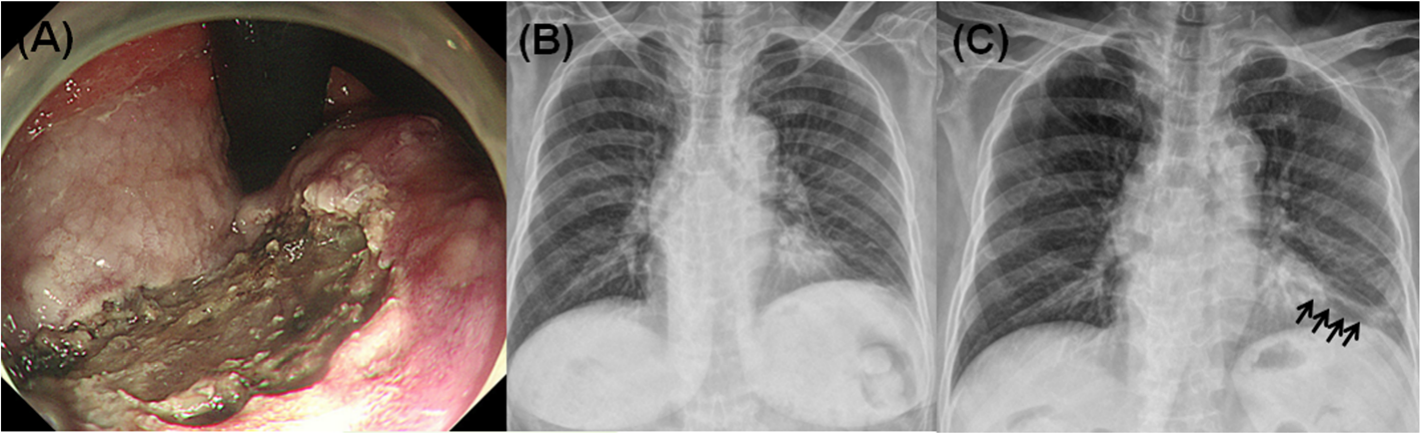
One example of atelectasis in an elderly patient (patient aged 75 years over who underwent endoscopic submucosal dissection). Photo of procedure a. X-ray before procedure b and 24 h after the procedure c
